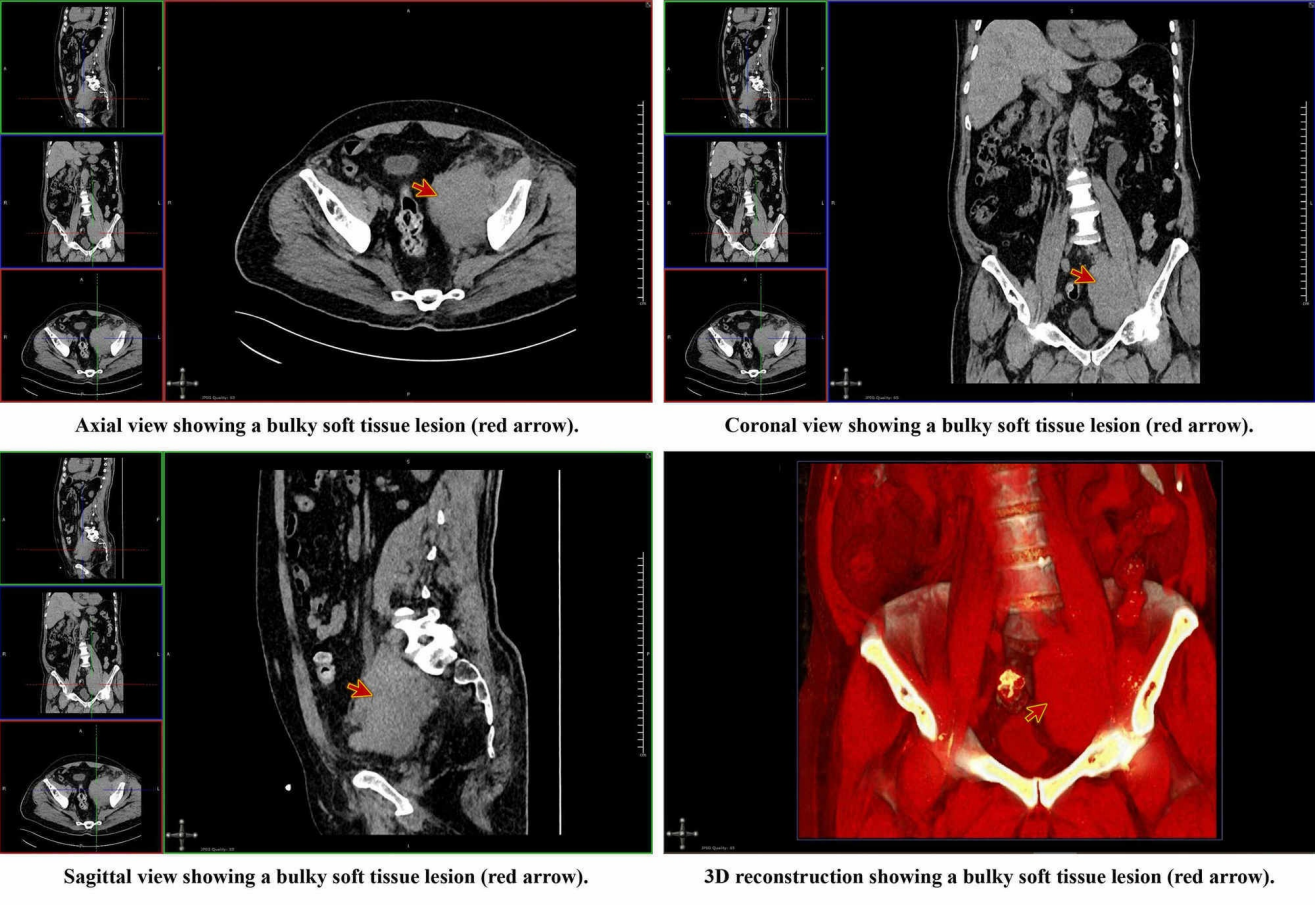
Axial, coronal, sagittal, and 3D reconstruction views of the CT of kidney, ureter, and bladder (CT KUB) CT: computed tomography
